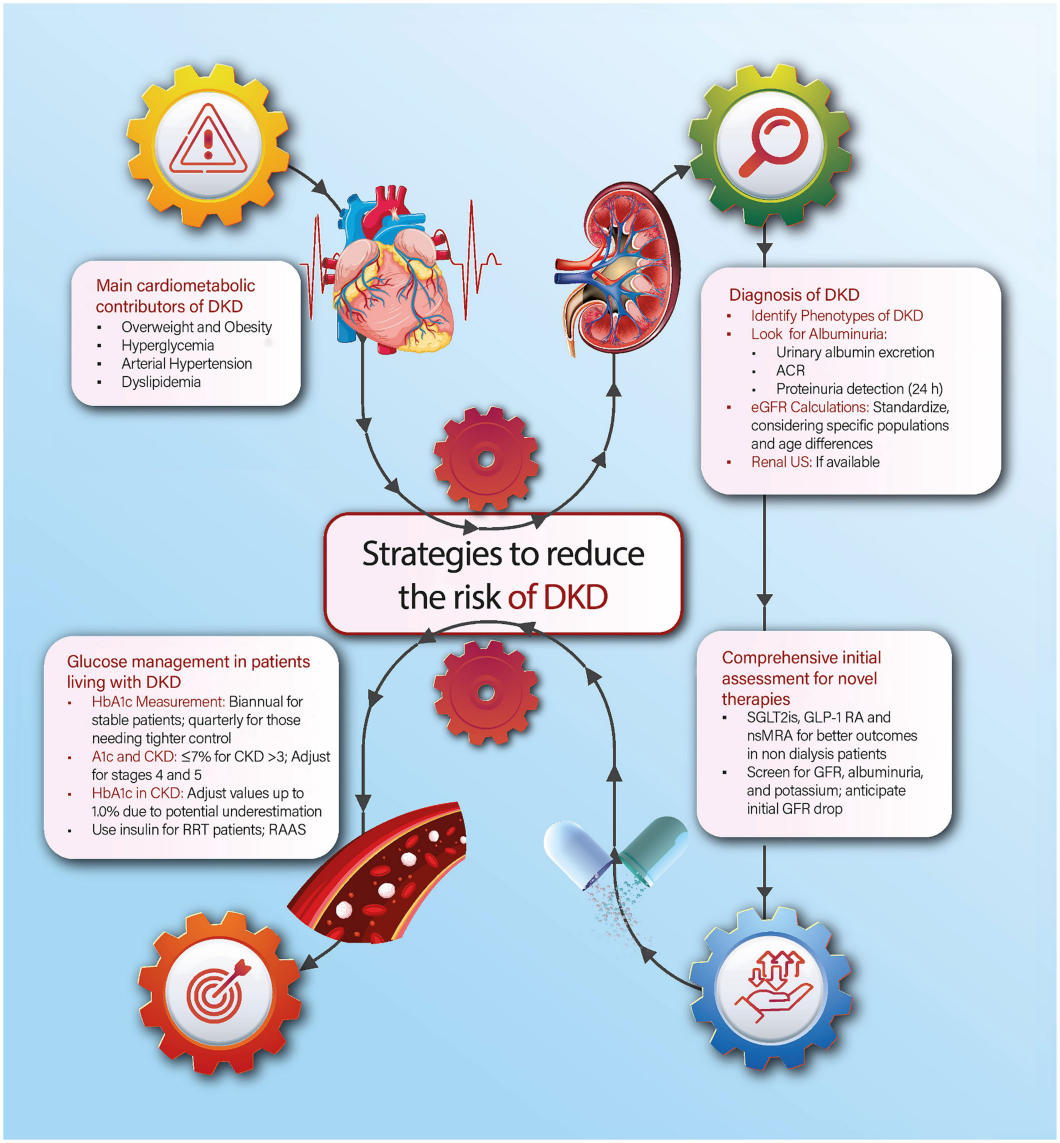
Summary of four strategies to reduce the burden of CKD in patients living with diabetes in the context of Mexico. A1c, Glycated hemoglobin; ACR, Albumin-to-Creatinine Ratio; CKD: Chronic Kidney Disease; DKD, Diabetic Kidney Disease; eGFR, Estimated Glomerular Filtration Rate; GLP-1 RA, Glucagon-Like Peptide-1 Receptor Agonists; nsMRA, Non-steroidal Mineralocorticoid Receptor Antagonists; RAAS, Renin-Angiotensin-Aldosterone System, RRT, Renal Replacement Therapy; SGLT2i, Sodium-Glucose Co-Transporter-2 Inhibitors; US, Ultrasound.
